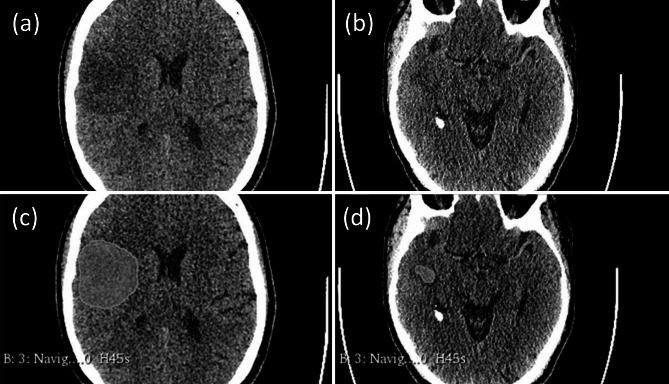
Preoperative native CT images (axial slice) of two patients with IDH-mutated (left images) and non-IDH-mutated (IDH wildtype) glioma (right images). Figures (a) and (b) show the CT images without the overlaid segmented areas. The areas marked in green in figures (c) and (d) show the corresponding semi-automatic segmentations with 3D Slicer. Left figures (a) + (c): Case with IDH mutation. 53-year-old patient with an IDH mutated astrocytoma located in the right insular region. Right figures (b) + (d): Case without IDH mutation. 59-year-old patient with a IDH wildtype glioma located in the right temporal lobe.
